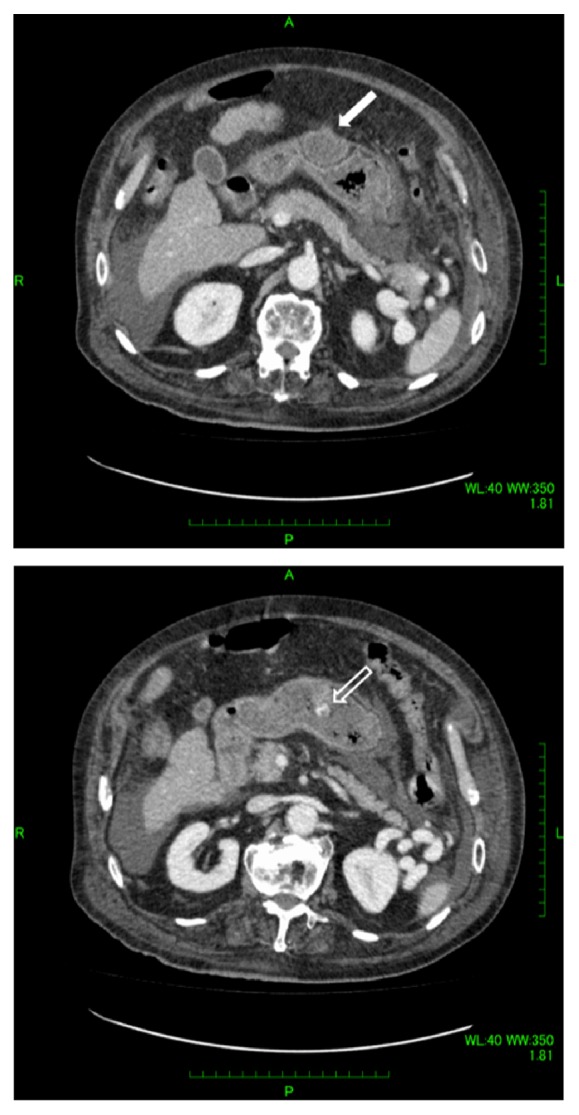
A contrast enhanced computed tomography scan demonstrated gastric extraluminal compression by hepatocellular carcinoma (arrow) and extravasation of contrast medium into the stomach (open arrow).
